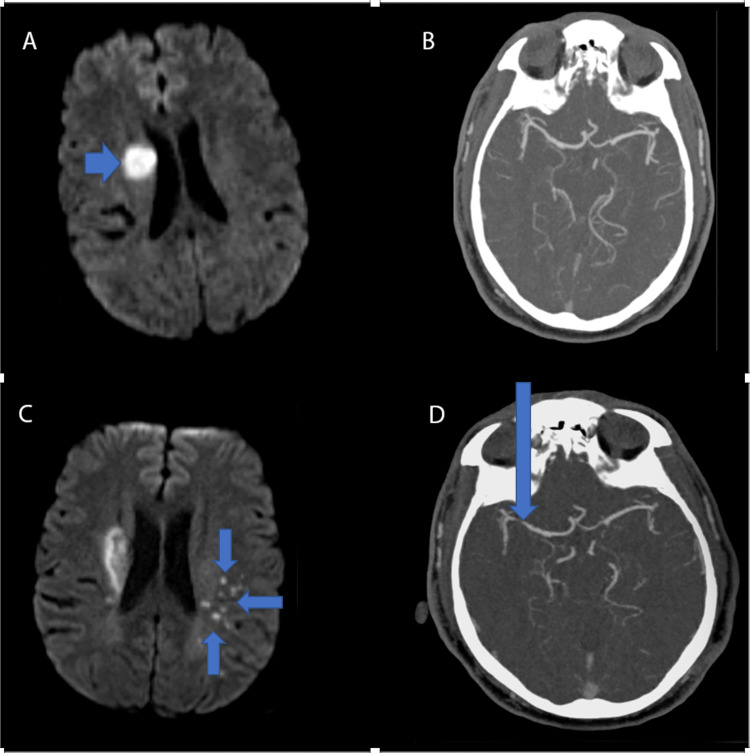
Neuroimaging findings for Case 1. (A) MRI Brain without contrast demonstrating right corona radiate ischemic stroke; (B) Initial CTA Head and Neck with contrast demonstrating normal findings; (C) MRI Brain without contrast demonstrating new, multiple, punctate infarcts in the left frontoparietal area; (D) CTA Head and Neck with contrast revealing new stenosis of the distal right M1. MRI: magnetic resonance imaging; CTA: computed tomography angiography
